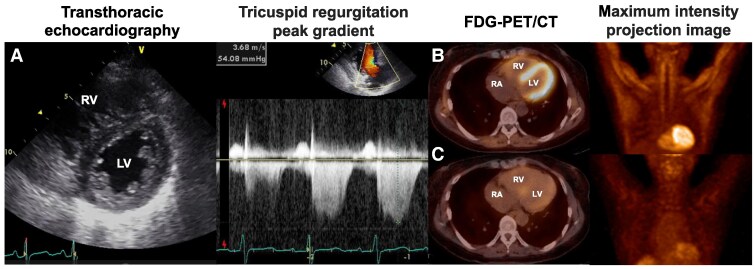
(*A*) Transthoracic echocardiography showing enlarged right ventricle with interventricular septal displacement towards the left ventricle and mild tricuspid regurgitation and estimated systolic pressure of 54 mmHg. (*B*) Increased glucose metabolism was noted in the biventricular free walls and skeletal muscles with glucose loading. (*C*) Increased glucose metabolism was not observed in the myocardium and skeletal muscles without glucose loading.
